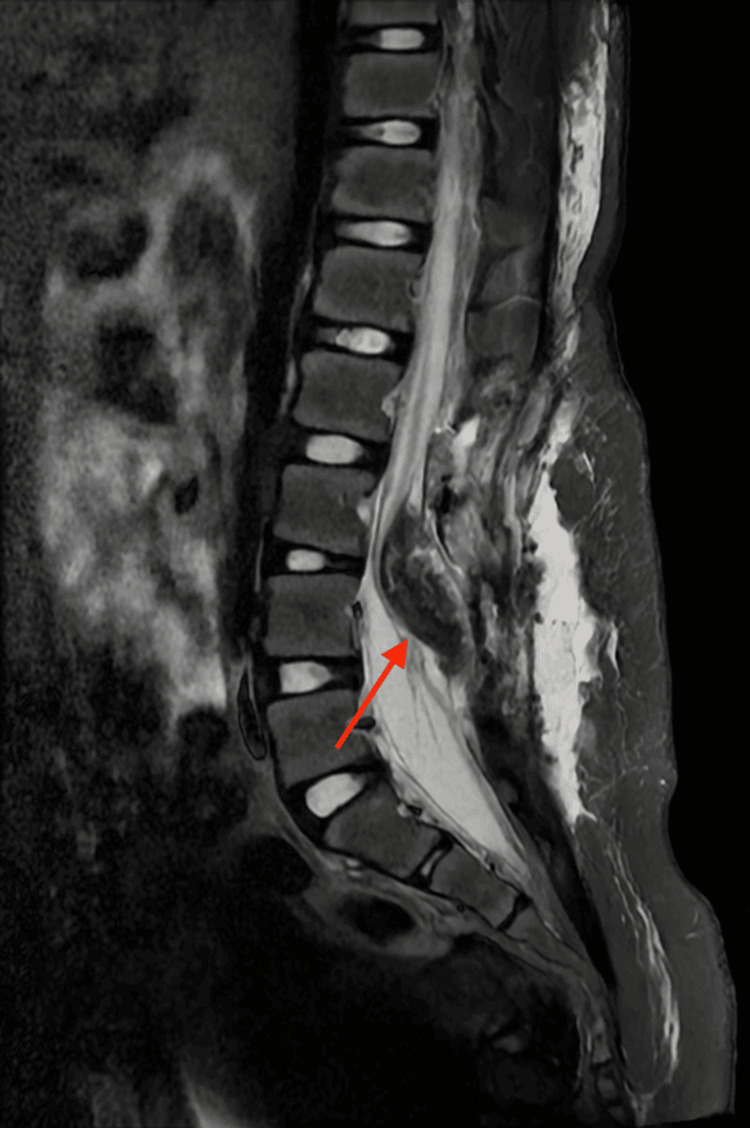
Sagittal lumbar magnetic resonance imaging Low-lying conus medullaris fused with hyperintense lipomatous tissue extending through a bony defect into the subcutaneous tissue, findings consistent with lipomyelomeningocele and tethered cord.
